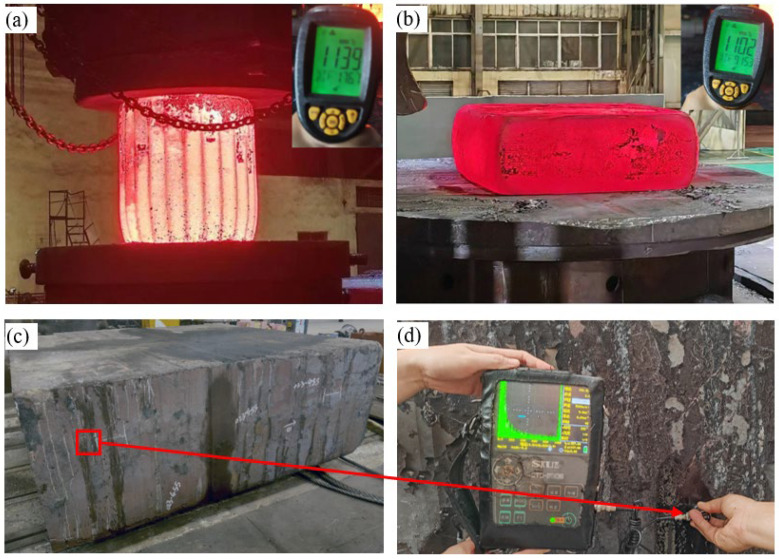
The 183 t forging of a novel Cr-Mo-V die steel: (a) upsetting, (b) forged product, (c) finished forging, and (d) ultrasonic testing.
